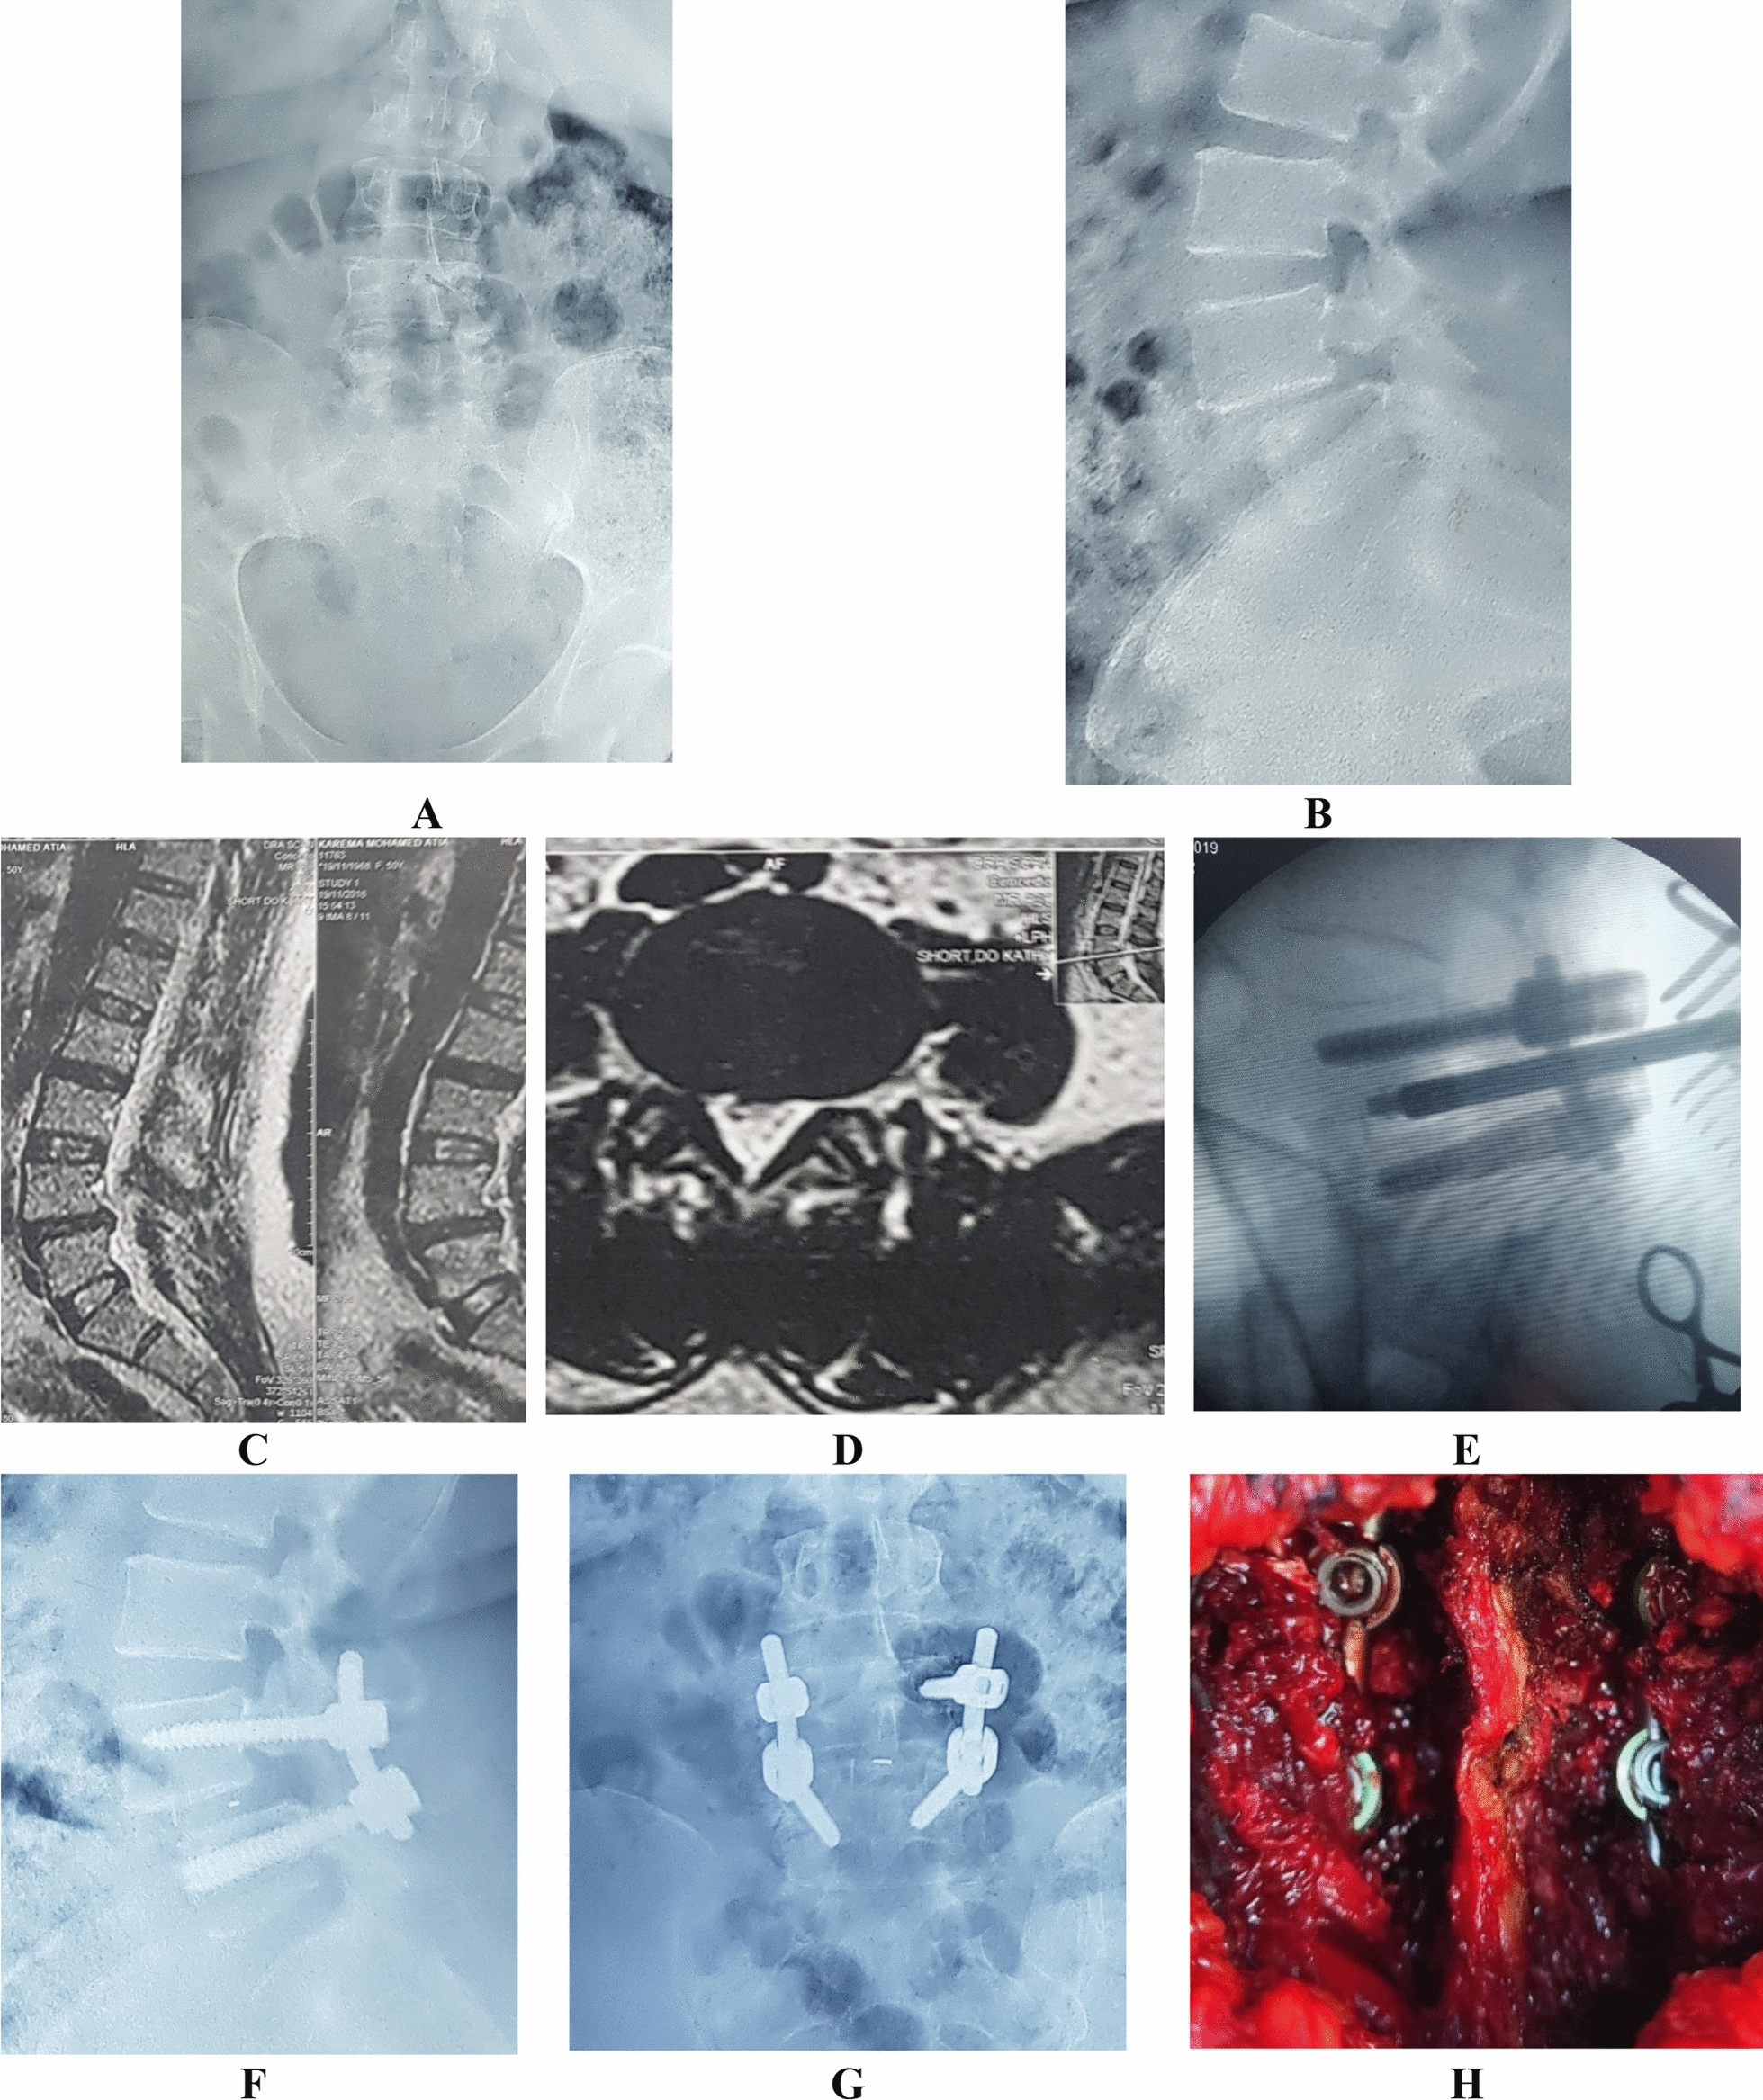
The open TLIF case, A and B anterior–posterior and lateral radiology of the slipped level, C and D MRI sagittal and axial cut, E intraoperative photo during insertion of the cage, F and G the final Anterior–posterior and lateral view of the operated level, and finally H: intra-operative photo of the operative field
